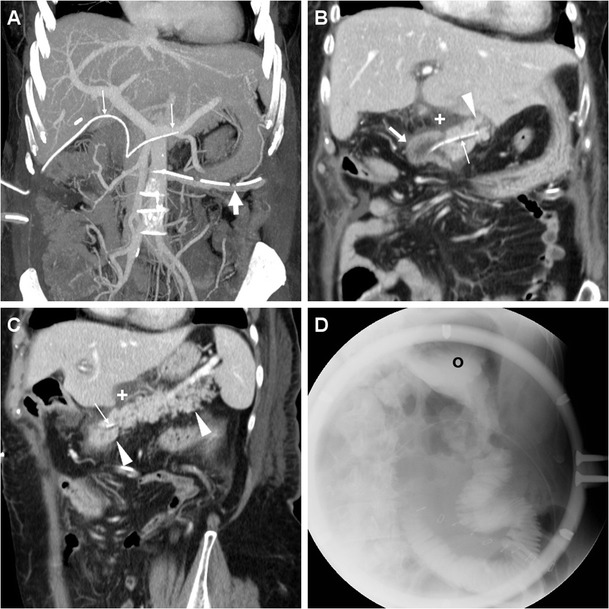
Expected CT findings following uncomplicated PD performed for malignant intraductal mucinous-papillary tumour of the pancreatic head in a 69-year-old woman. Coronal maximum intensity projection (MIP) reconstruction (a) showed presence of a left-sided abdominal drainage tube (*thick arrow*) and of an externally draining trans-anastomotic stent (*thin arrows*). Focused coronal (b) and oblique-coronal (c) contrast-enhanced images showed minimal fluid (+) abutting the PJS, normal appearance of the PR (*arrowheads*) and MJL (*arrows*). In the same patient, fluoroscopy (d) showed normal flow of oral contrast medium (CM) from the gastric remnant (o) through the GJS
